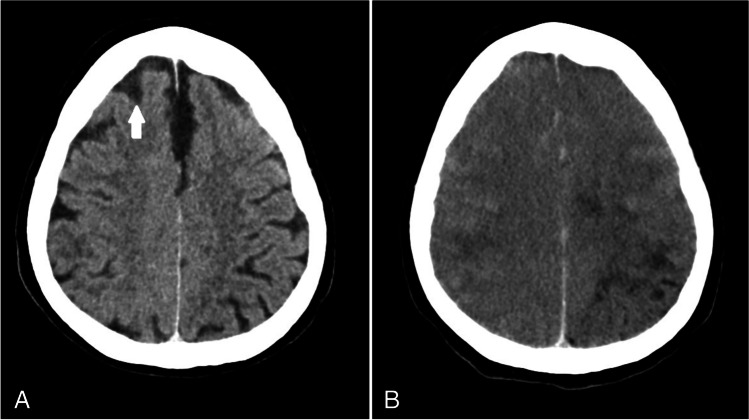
Case 2. Non-contrast brain CT performed directly after percutaneous coronary intervention (A) showed increased density of the CSF (arrow). Follow-up CT 7 h later (B) demonstrated progressive brain swelling
